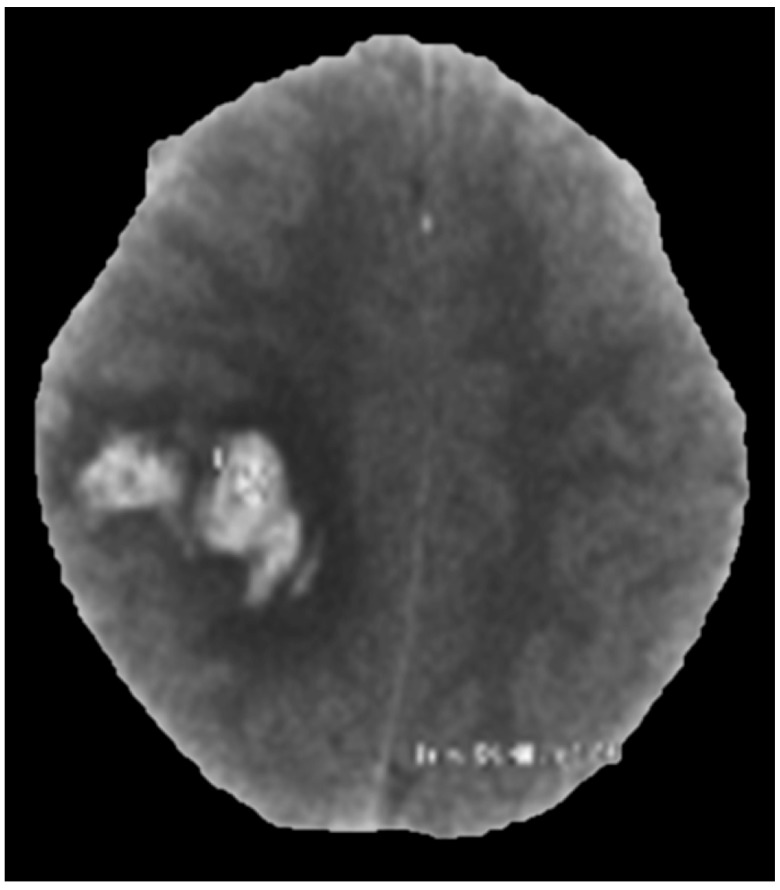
CT scan head showing a right high parietal hemorrhagic infarct with surrounding edema.
